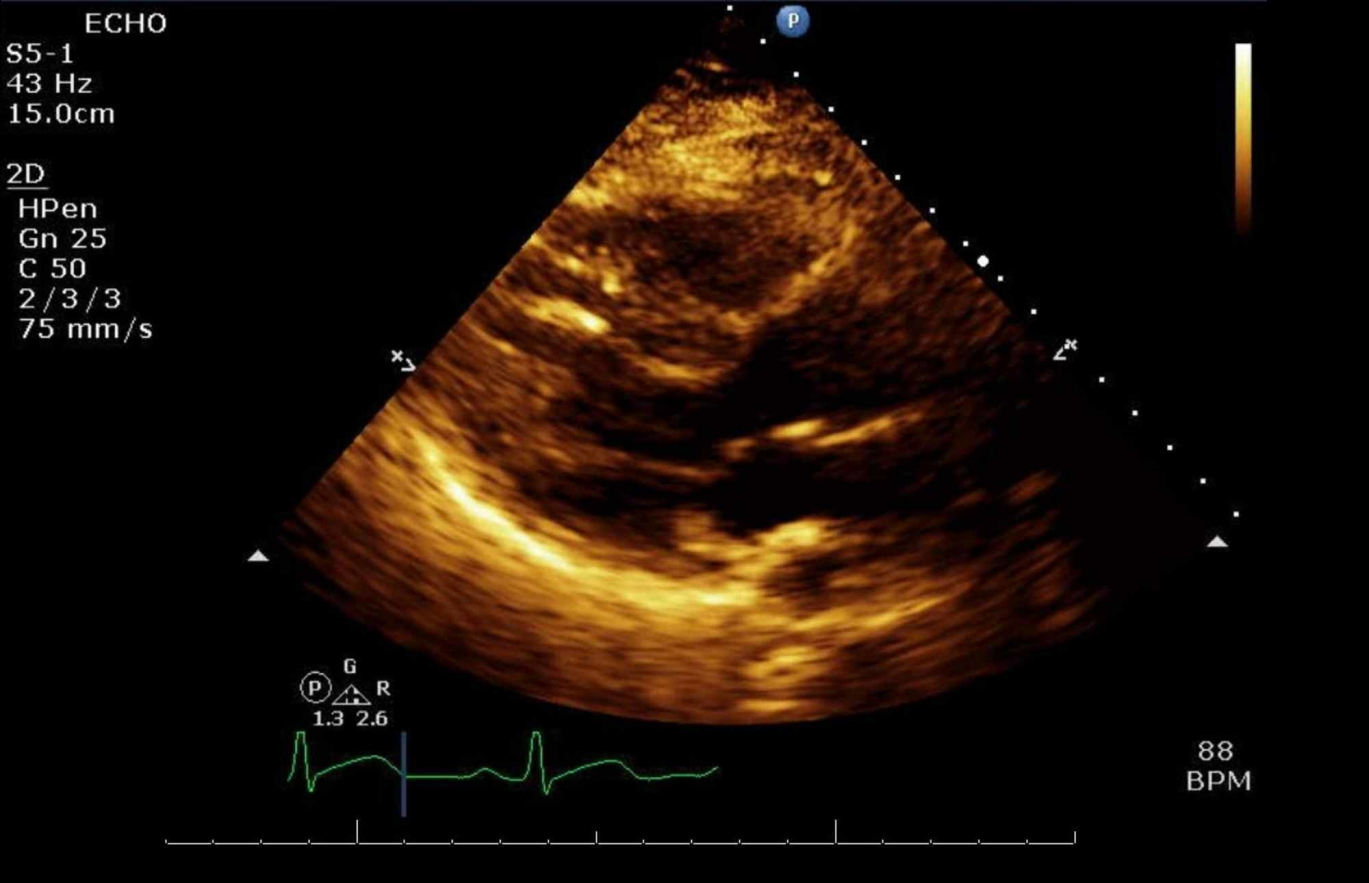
An unremarkable trans-thoracic echocardiogram (TTE)
